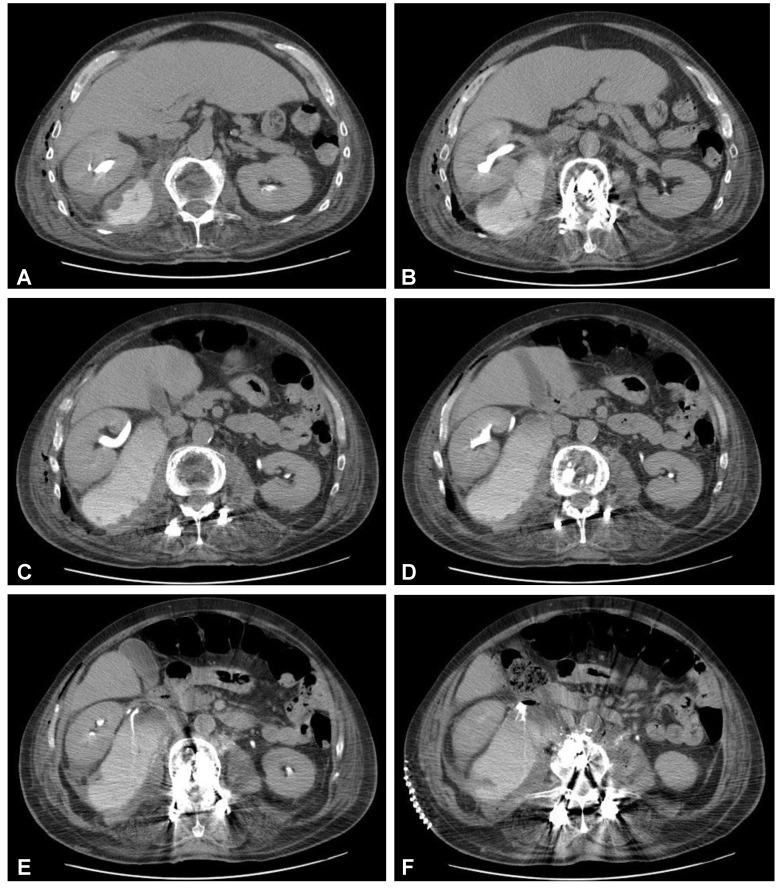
Postoperative axial CT scans (contrast enhanced delayed phase) demonstrating evidence of post-traumatic right ureteral injury, urine leakage, and hydronephroureter (A–F).
